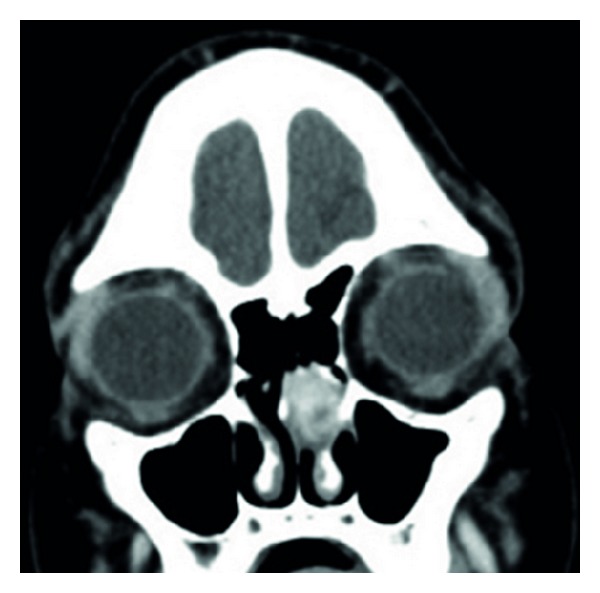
A head CT with contrast demonstrating an LCH in the left nasal cavity.
